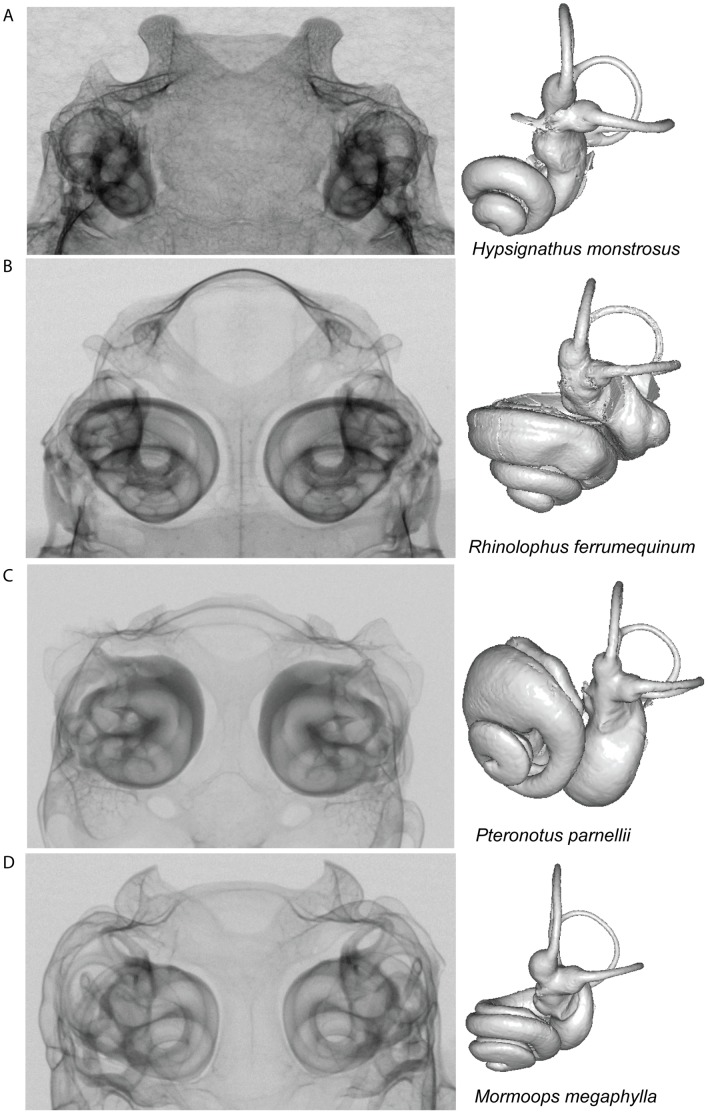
X-ray projections through the posterior portion of bat skulls containing the bony labyrinth, in the ventral-dorsal plane. The corresponding reconstructed inner ear volumes are shown on the right of the panels; these inner ears are orientated so that the lateral semicircular canals are maximally aligned in the horizontal plane, allowing differences in cochlear orientation to be compared across species. Echolocation mode, species and family information are as follows: (A) the non-echolocating Old World fruit bat *Hypsignathus monstrosus* (1.3518) (Pteropodidae); (B) the Old World CF bat *Rhinolophus ferrumequinum* (58.20697) (Rhinolophidae); (C) the New World CF bat *Pteronotus parnellii* (5.21236) (Mormoopidae) and (D) *Mormoops megaphylla* (2.1603) (Mormoopidae) which utilizes narrowband multi-harmonic signals.
